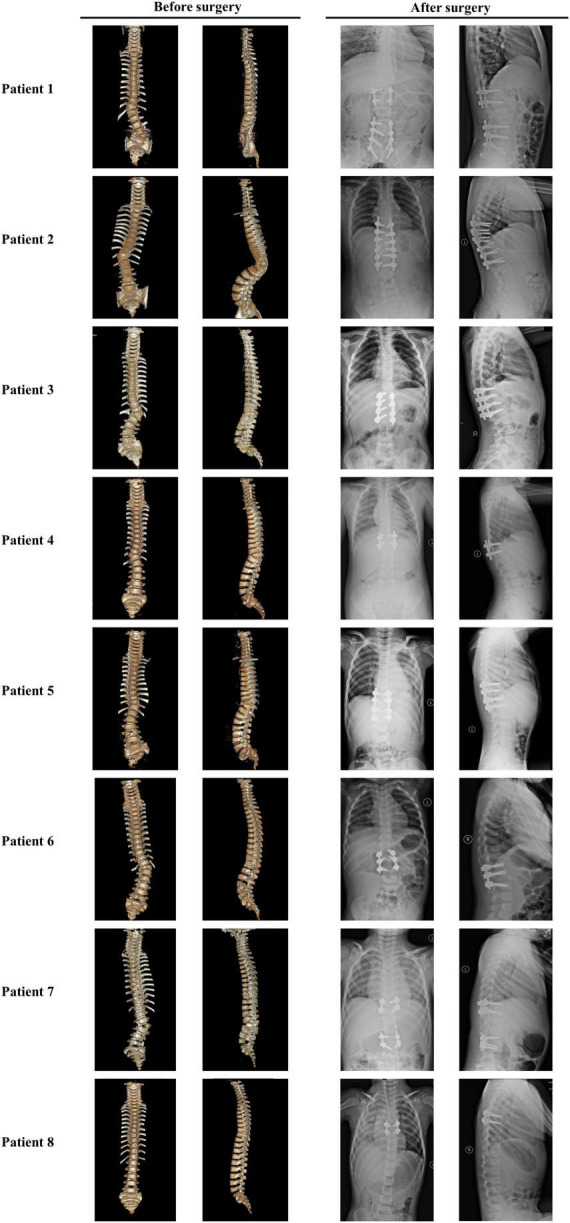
Images of patients with TACS before and after surgeries suggested well outcomes. Patient 1 had hemivertebrae at T12 and L5 with the Cobb angle of 26°; after surgery, the Cobb angle was reduced to 2°. Patient 2 had hemivertebrae at T11 with the Cobb angle of 53°; after surgery, the Cobb angle was reduced to 87°. Patient 3 had hemivertebrae at T12 and butterfly vertebrae at T11 with the Cobb angle of 50°; after surgery, the Cobb angle was reduced to 5°. Patient 4 had hemivertebrae at T11 with the Cobb angle of 41°; after surgery, the Cobb angle was reduced to 0°. Patient 5 had hemivertebrae at T11 and butterfly vertebrae at L4 and S3 with the Cobb angle of 30°; after surgery, the Cobb angle was reduced to 3°. Patient 6 had hemivertebrae at T12 and butterfly vertebrae at L5 with the Cobb angle of 46°; after surgery, the Cobb angle was reduced to 6°. Patient 7 had unsegmented hemivertebrae at T10 and L3 and butterfly vertebrae at C7 and T9 with the Cobb angle of 22°; after surgery, the Cobb angle was reduced to 3°. Patient 8 had hemivertebrae at T8 with the Cobb angle of 26°; after surgery, the Cobb angle was reduced to 4°. Images before and after surgeries were obtained by CT and X-ray, respectively. TACS, *TBX6*-associated congenital scoliosis.
